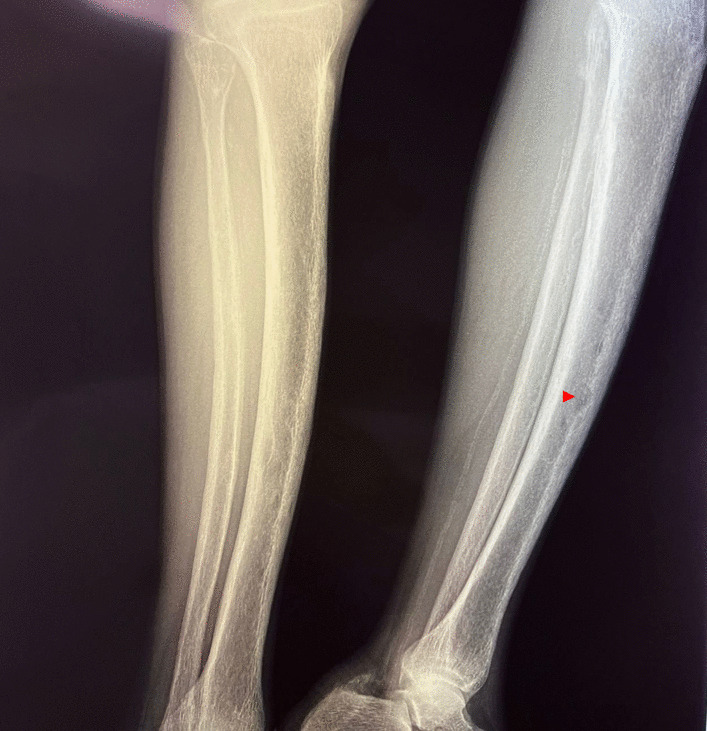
X-ray of the tibia and fibula showed lytic lesion (arrowhead)
